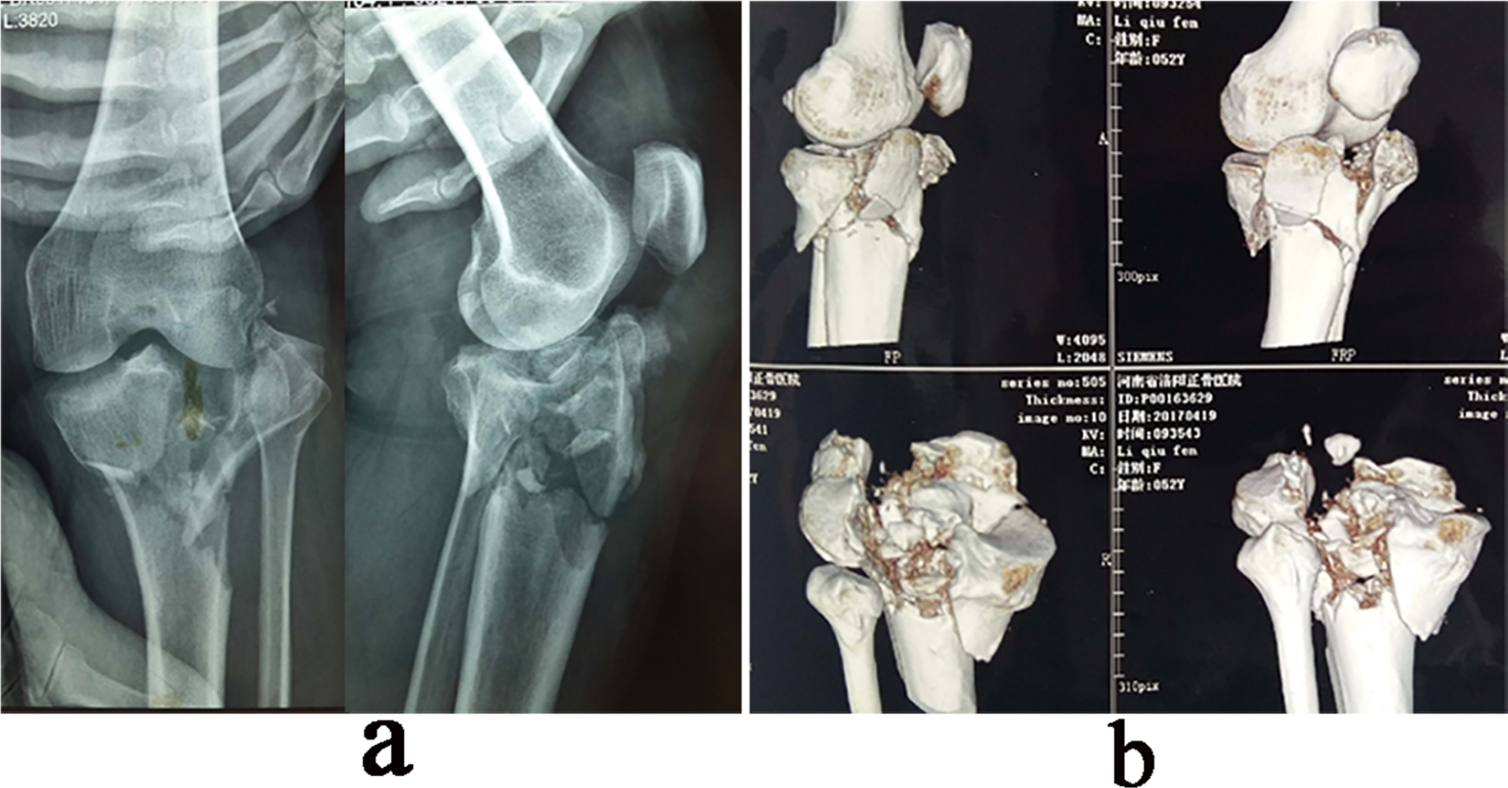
A 45-year-old female patient with an old and complex tibial plateau fracture. (a) Preoperative X-ray film showing the left tibial comminuted fracture (Schatzker type V). (b) Three-dimensional CT images of the fracture showing a complex tibial plateau fracture.
